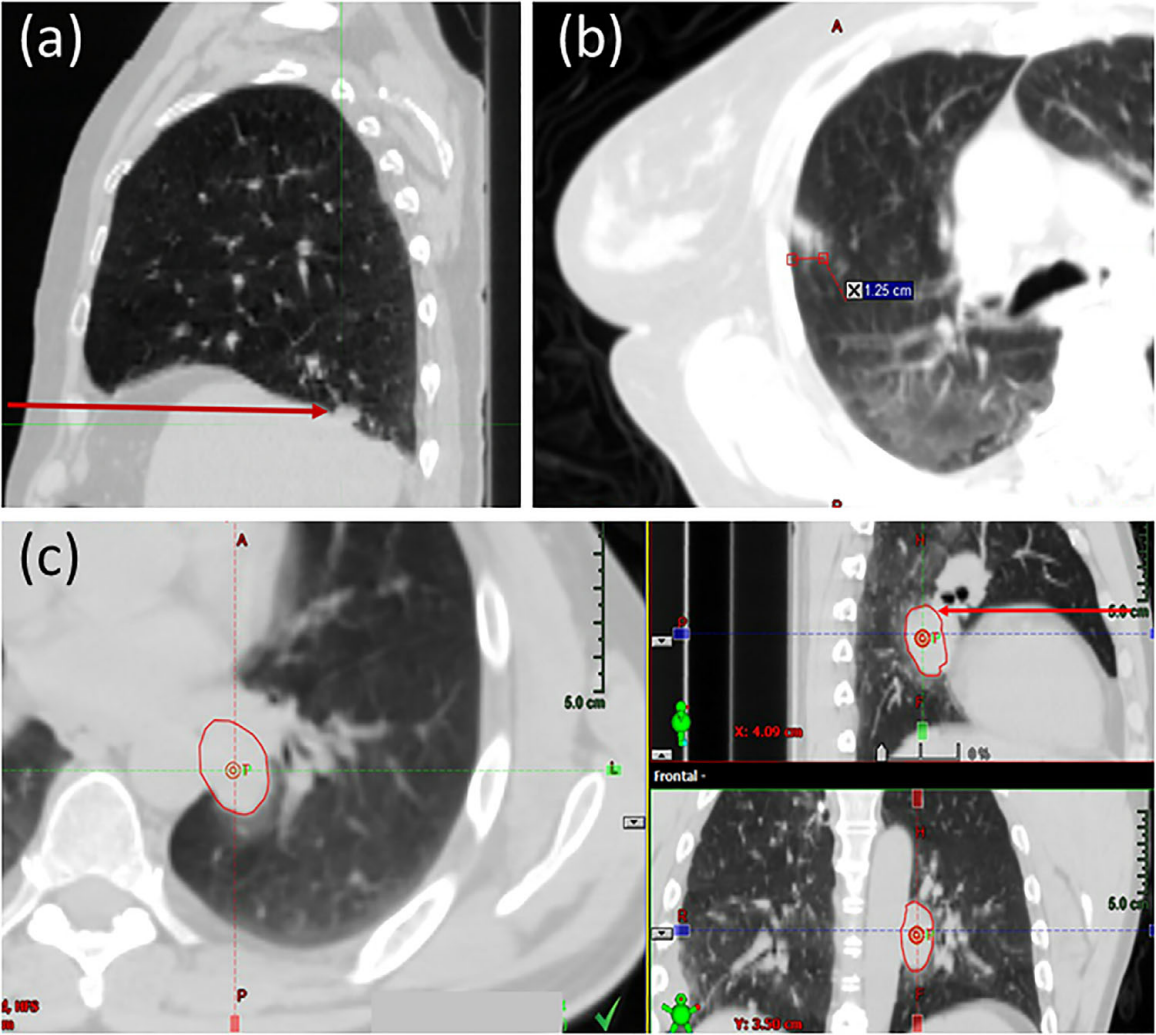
Example cases where thoracic tumors are not visible in fluoroscopic imaging. (a) Sagittal CT image showing tumor located posterior to the diaphragm and inferior to the apex of the diaphragm, such that diaphragm will obscure tumor visualization in an anterior–posterior projection. (b) Axial CT image showing a diffuse tumor, or ground glass object, with signal that is too faint to be observed in fluoroscopy. (c) Axial, sagittal, and coronal CT images of a tumor that is located posterior to mediastinal soft tissue, such as the heart
